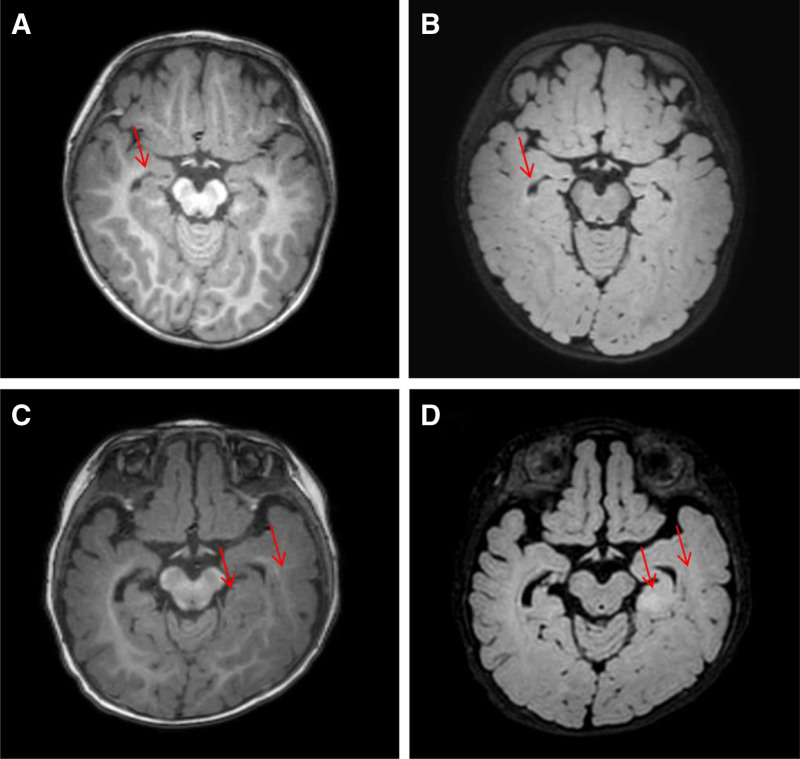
Brain MRI images of the 2 patients. Brain MRI of Patient 1 showing a slightly smaller right hippocampus on T1-weighted imaging (A) and a fluid-attenuated inversion recovery (FLAIR) sequence (B). Brain MRI of Patient 2 showing swelling of the left temporal lobe with abnormal signal intensity suggestive of FCD and an abnormal signal in the left hippocampus on T1-weighted imaging (C) and fluid-attenuated inversion recovery (FLAIR) sequence (D). FCD = focal cortical dysplasia, MRI = magnetic resonance imaging.
